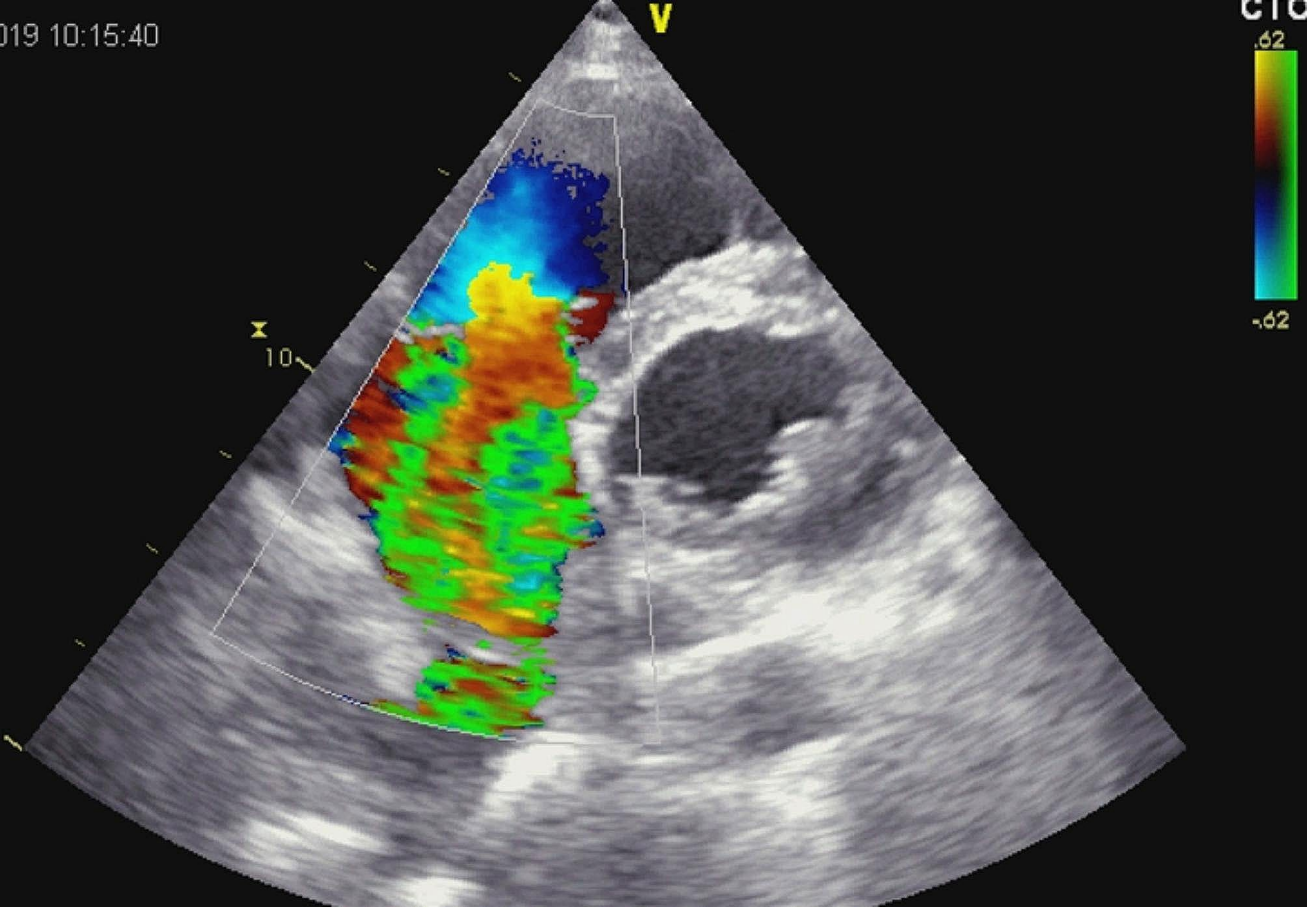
Massive tricuspid regurgitation, shown by transthoracic colour doppler (parasternal short axis view)
